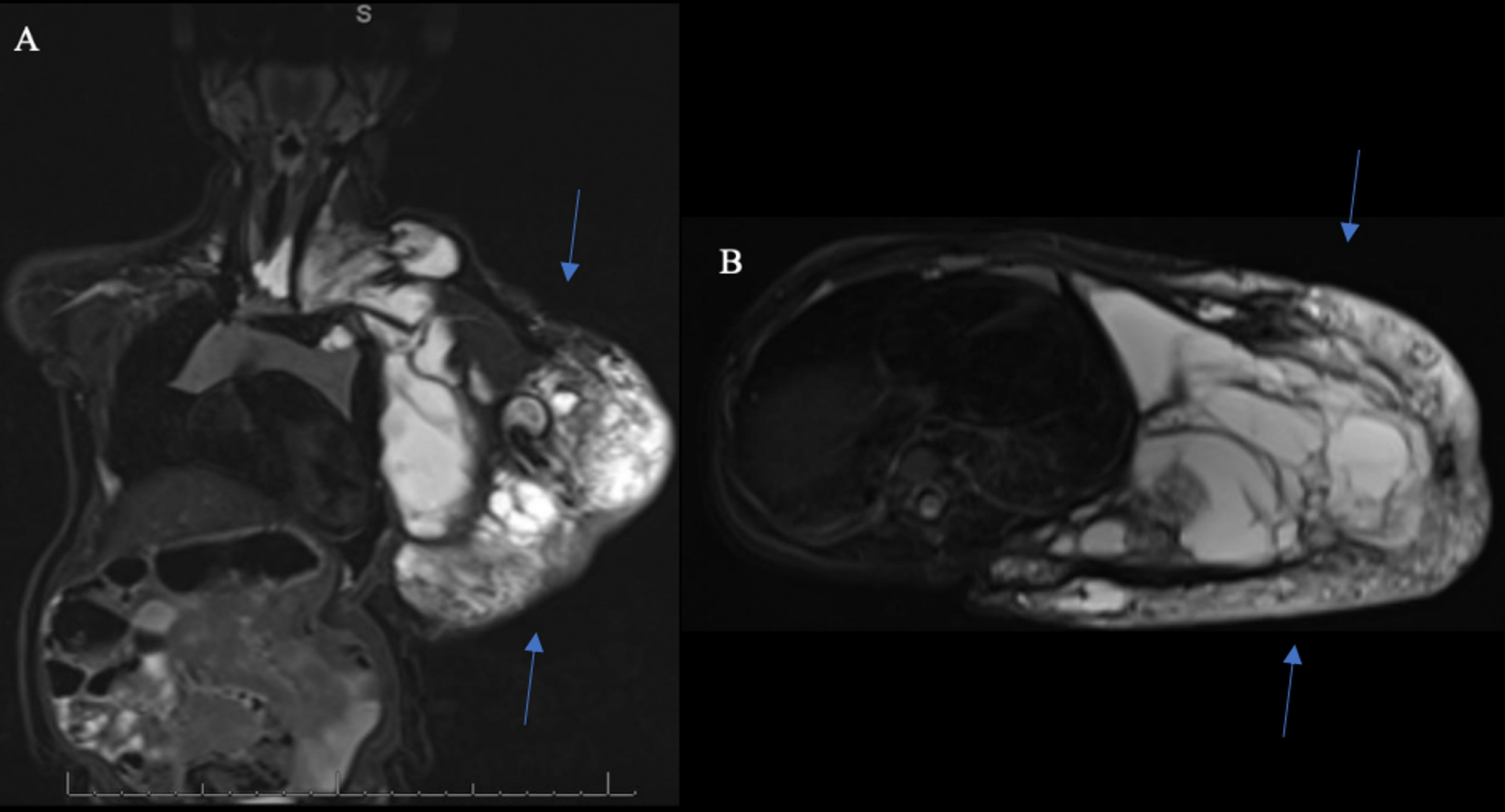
Imaging of Venolymphatic Malformation T2-weighted spin-echo images with fat-saturation in the coronal (A) and axial (B) planes show a large, spectated, multispatial lesion with fluid-fluid levels consistent with a venolymphatic malformation. The lesion extends from the left anterior chest to the back across the supraclavicular and suprascapular regions. The left lateral chest wall including the axillary regions contain a large bulk of the lesion.
